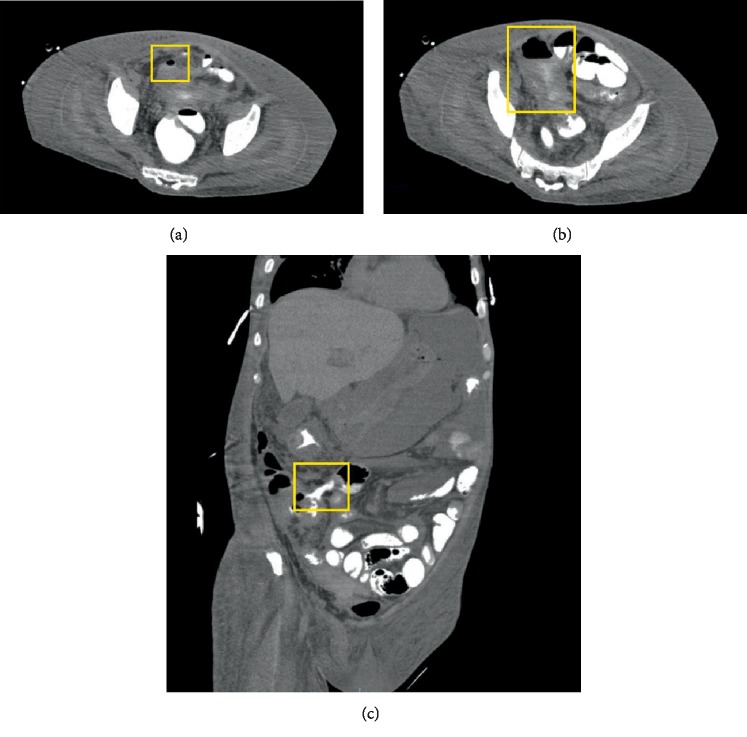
CT scan images of significant findings on hospitalization including (a) Free air in the pelvis. (b) Free air with leaked contrast in the peritoneal space. (c) An enterocolic fistula. These findings determined the decision for exploratory laparotomy.
